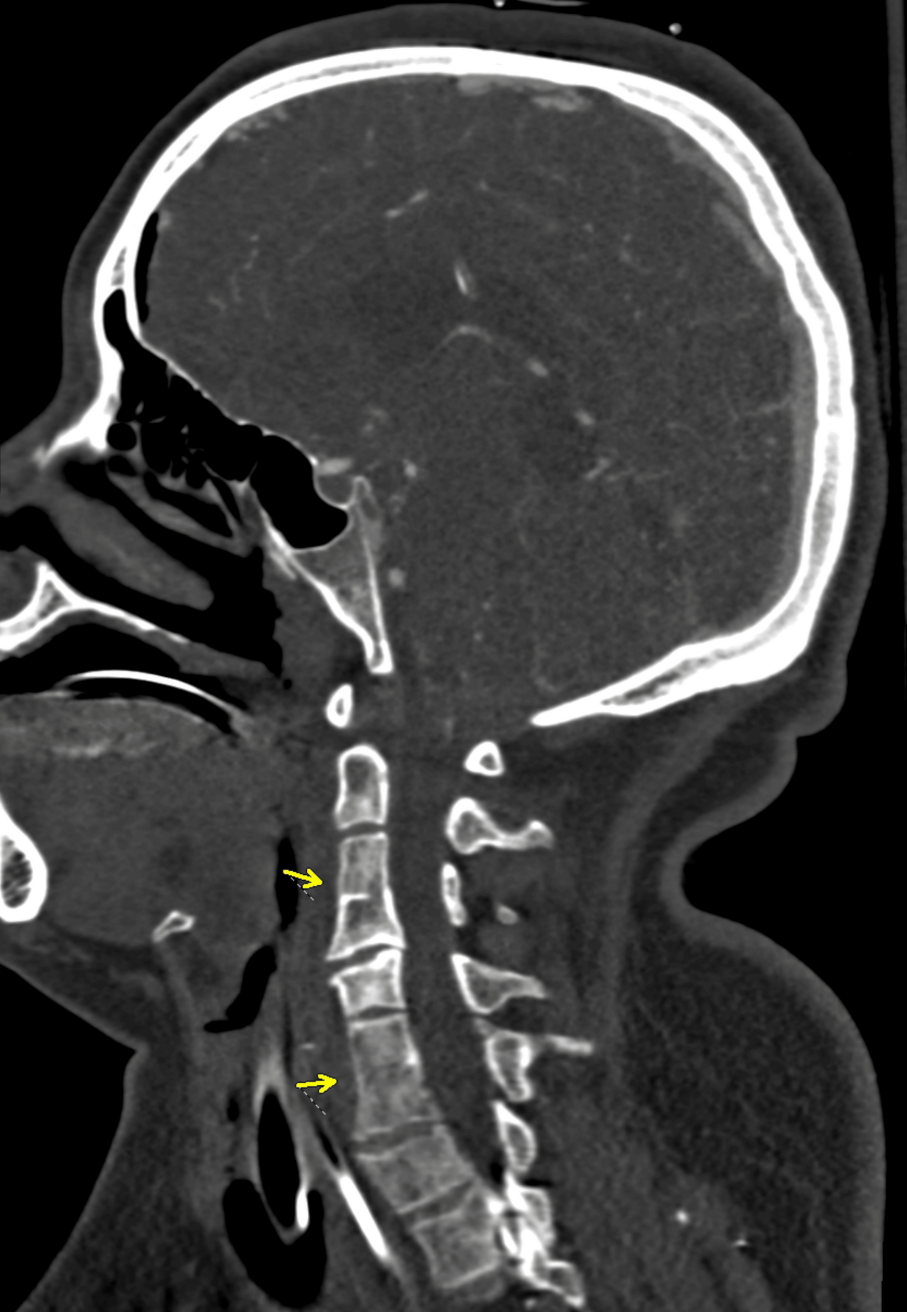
Sagittal CTA of the cervical spine demonstrating congenital C3-C4 and C6-C7 fusion, radiographic features suggestive of Klippel-Feil syndrome (yellow arrows). CTA = computed tomography angiography
